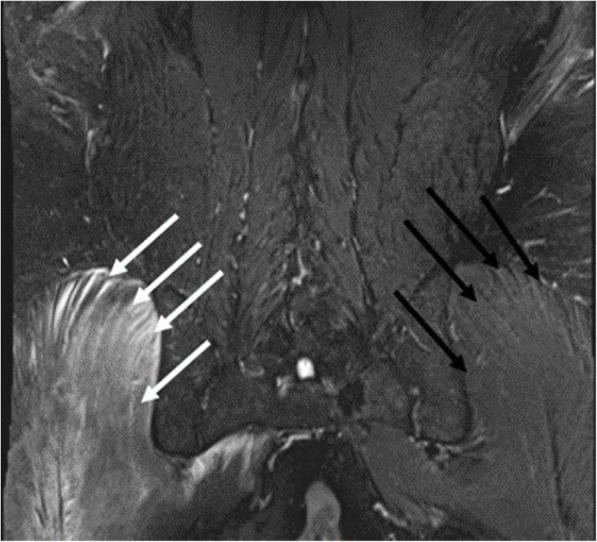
Patient’s MRI postoperatively of the lumbosacral region demonstrating hyperintense signaling from the right gluteal muscles (white arrows), please notice the difference between it and the normal left gluteal muscles (black arrows)
